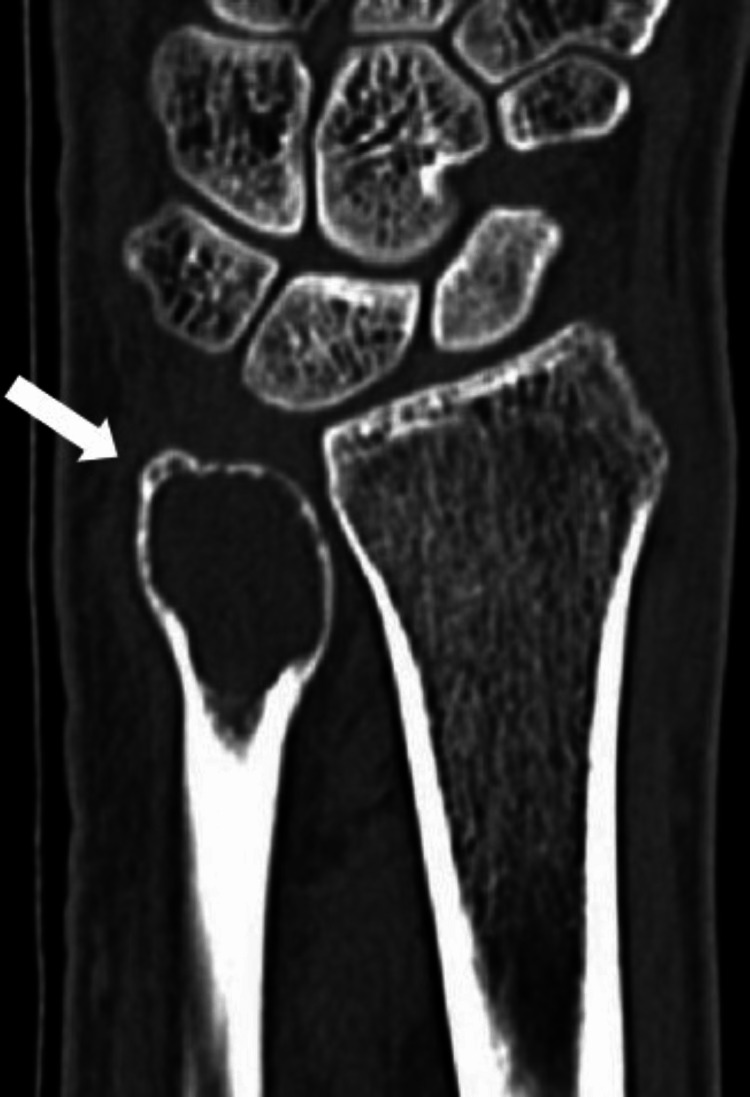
Computed tomogram of the left wrist CT scan of left wrist showing a thin and protruded cortex but no destruction of the cortex of the distal ulna
